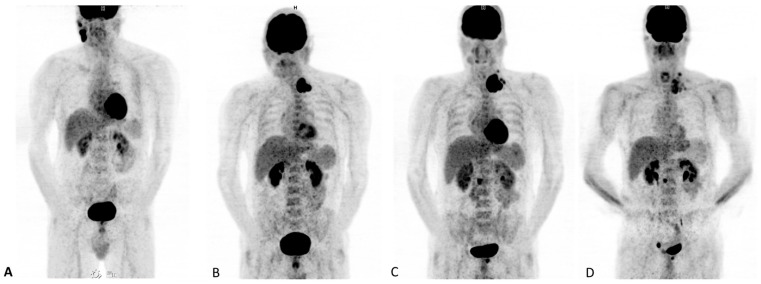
48-year-old man with right temporal melanoma operated in 2016. (A) Tumoral progression in laterocervical lymphadenopathies and intraparotid adenopathy in the right side. (B) [^18^F]FDG PET/CT 3 months later, under treatment with Dabrafenib and Trimetinib, shows a complete response in the right side with lymphadenopathic progression on the left side, where a new conglomerate is identified. (C) Control after one month shows lymphadenopathic progression and two new lesions in lumbar spine and pubis. (D) After 4 cycles of Nivolumab and Ipilimumab, there is improvement in cervical lymph node involvement, while bone progression is observed in pelvis. This evolution suggests dissociated response.
